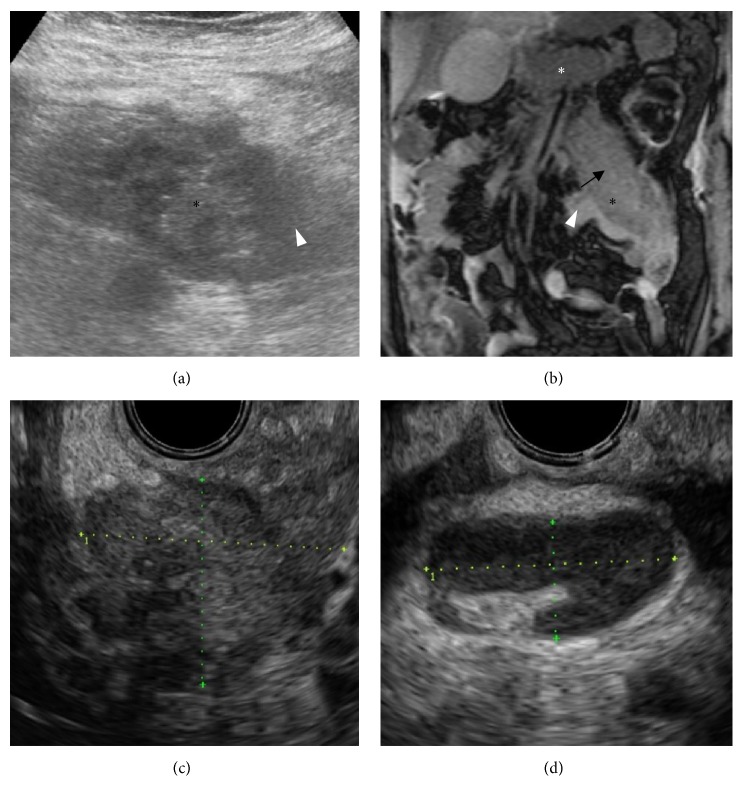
(a) Ultrasound image of the mesenteric soft tissue mass* (black asterisks) *with surrounding inflammatory changes* (white arrowhead)*. (b) Coronal TRUFI image depicting the soft tissue mass with signal characteristics according to the orthotopic pancreas* (white asterisk)*. Surrounding, edematous inflammatory changes* (white arrowhead)* and a possible central duct* (black arrow)*. (c) EUS image of the hypoechoic mesenteric soft tissue mass, in the transversal plane measuring approximately 3 × 4 cm. (d). EUS image of an enlarged mesenteric lymph node, with a short axis of 9 mm, demonstrating no suspicious morphological features.
